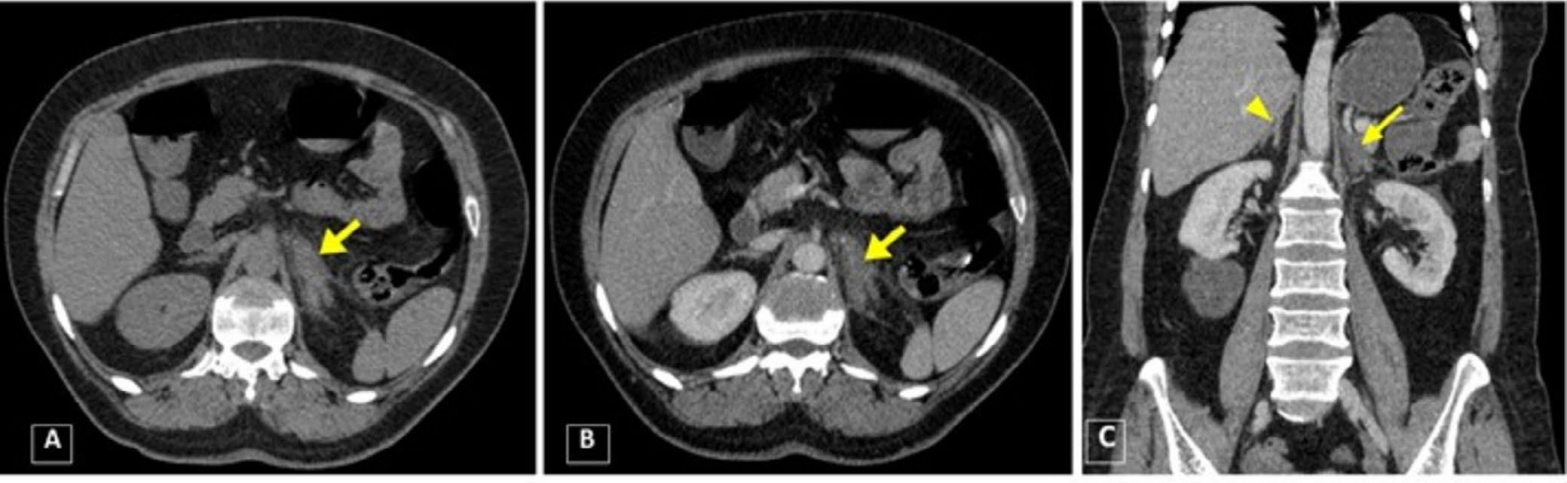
Axial computed tomography: (A) Non‐contrast‐enhanced CT scan (NECT) demonstrated asymmetry and bulging in the left adrenal gland (*arrow*), with no evidence of hyperdensity suggestive of hemorrhage. Furthermore, fat stranding was seen in the left peri‐adrenal space. (B) In the contrast‐enhanced CT scan (CECT), the left adrenal gland (*arrow*) was hypoattenuating with no evidence of enhancement. (C) In the coronal view, the arrow demonstrates that the left adrenal gland is bulged with no enhancement, in contrast to the normal appearance of the right adrenal gland (*arrowhead*). Additionally, a rim of perinephric fluid is noted around the left kidney.
